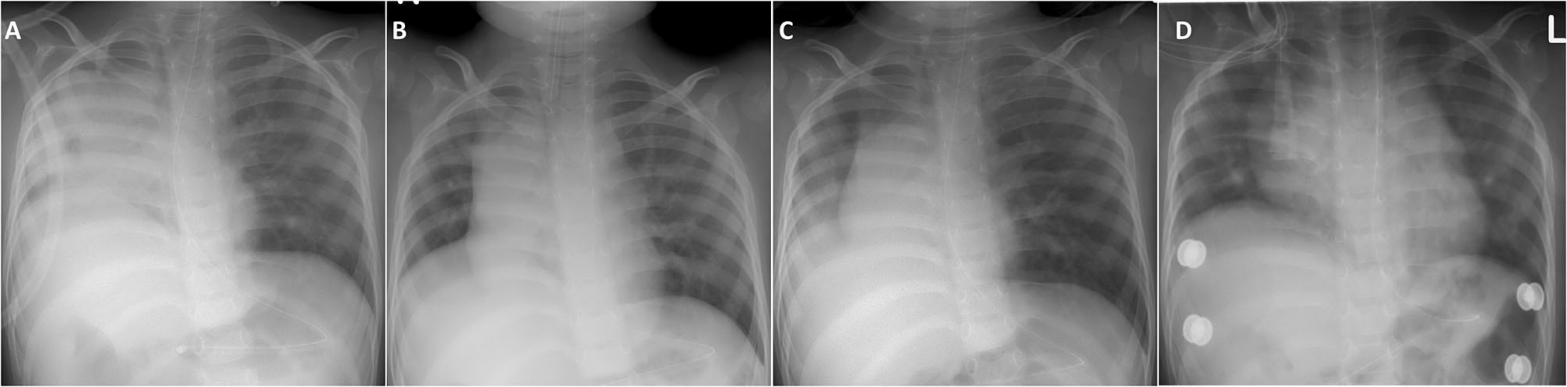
Demonstration of chest X-ray in a toddler with congenital myopathy who immediately received NIV and MI-E for post-extubation respiratory support. (A) Previously failed extubation in another hospital was related to frequent right lung atelectasis and mucus plugging developing soon after extubation. (B) In our hospital, appropriate expansion of both lungs were noted before extubation. (C) Day 2 post-extubation showed mild right lung infiltration without atelectasis. (D) Discharge from PICU on day 7 post-extubation showed re-expansion of both lungs.
